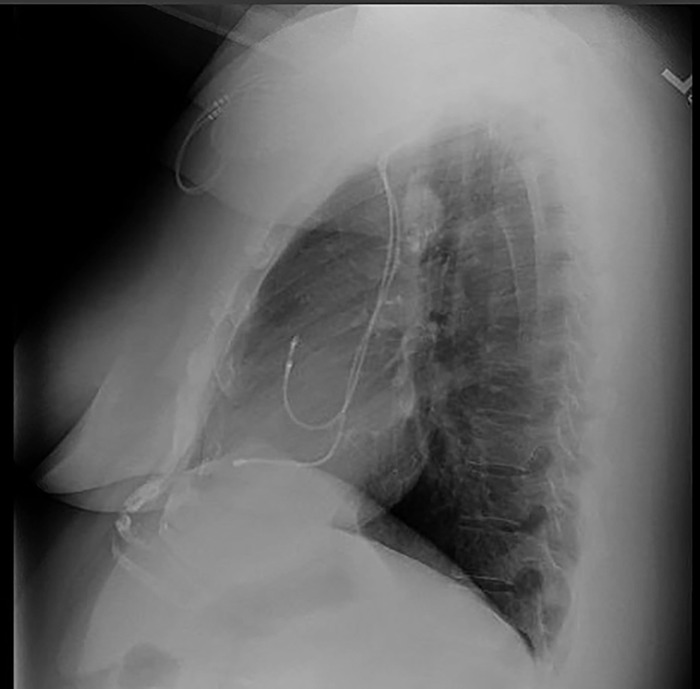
Preoperative chest x-ray lateral view shows the paratracheal mass.
